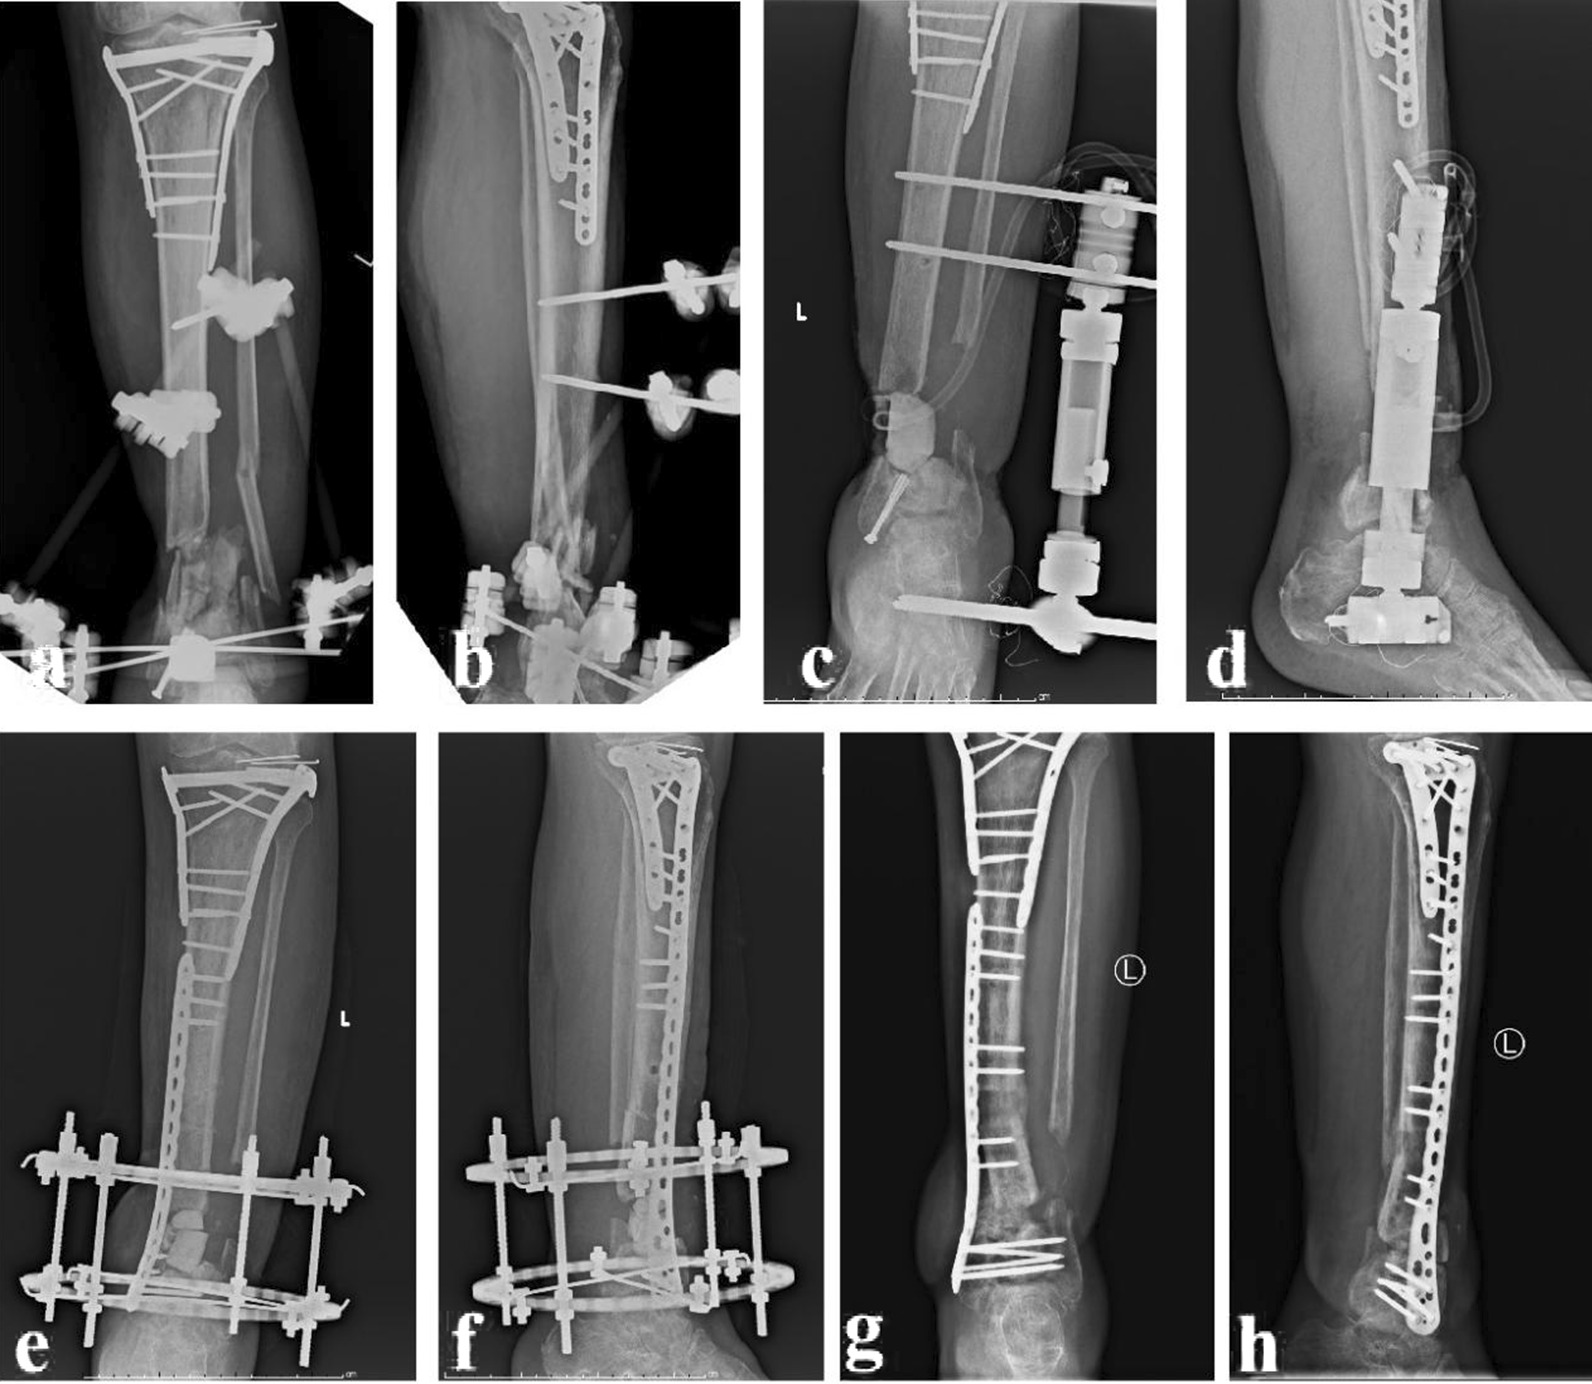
A 49-year-old male was diagnosed as osteomyelitis of distal tibia and treated with the ACSLHT technique. a and b X-rays before removal of all dead bones, c and d after debridement, 6.5 cm of distal tibial defects was observed, e and f this patient was treated with the ACSLHT technique, and X-rays showed the bone transport process, g and h X-rays after removal of the Ilizarov external fixator. ACSLHT stands for antibiotic calcium sulfate-loaded hybrid transport
